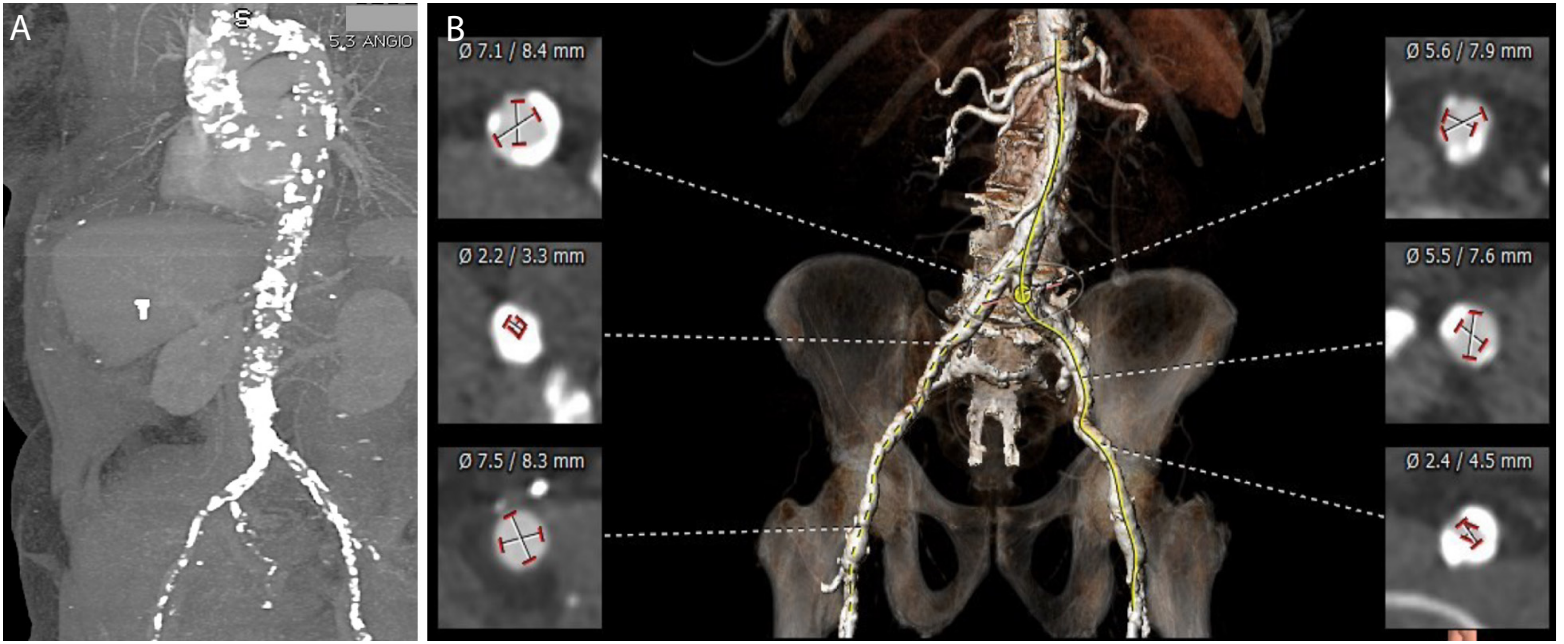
Computed tomography angiography reconstruction showing extensive aorta calcification (A) and important peripheral artery disease with circumferential calcification precluding transfemoral access (B).
